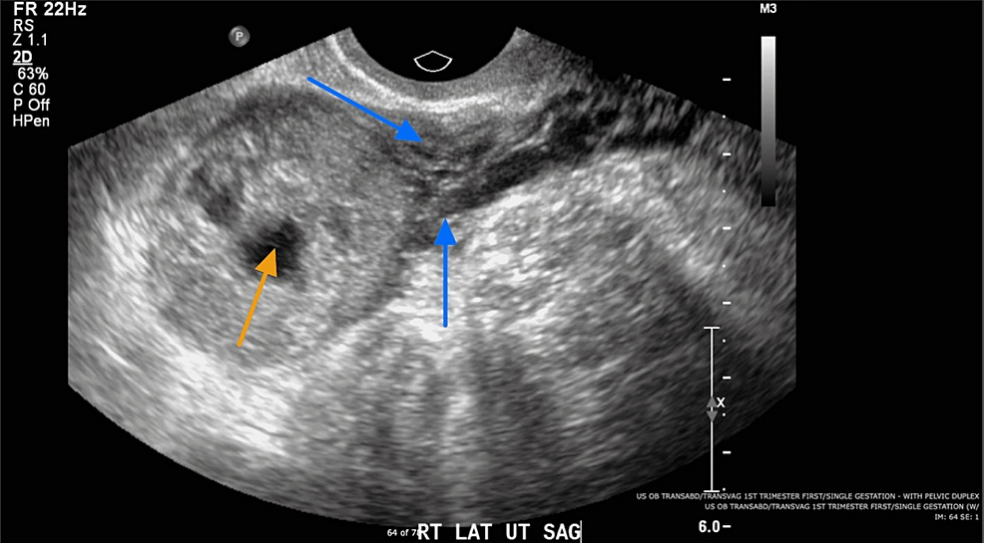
Transvaginal ultrasound indicating echogenic structure in the right lateral aspect of the uterine fundus. The central cystic component is indicated with the orange arrow. Blue arrows highlight a hypo-echoic myometrial band between the endometrial echos and the gestational sac.
